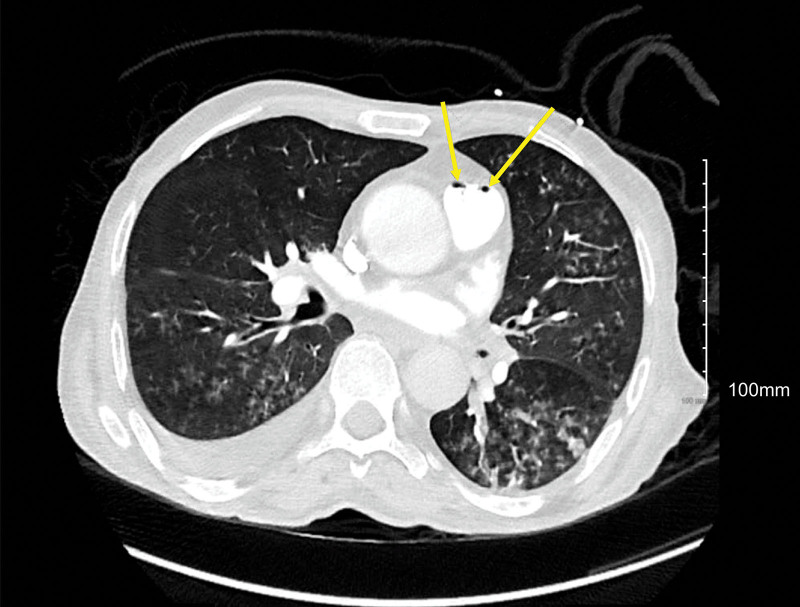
Chest CT scan showing air bubbles in the pulmonary trunk. CT = computed tomography.
